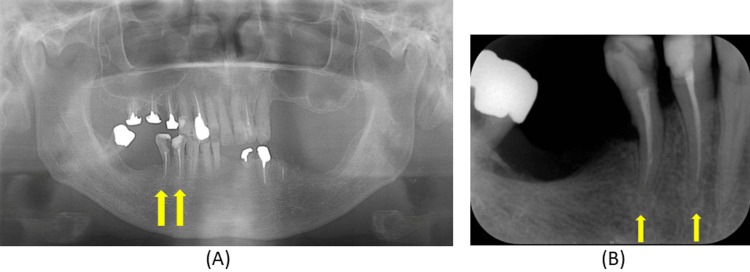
Radiographic findings at the initial examination (A) Panoramic radiographic findings; (B) Dental radiographic findings. These images provide baseline information on abutment tooth condition and help assess structural factors relevant to clasp engagement and long‑term prosthesis stability.
